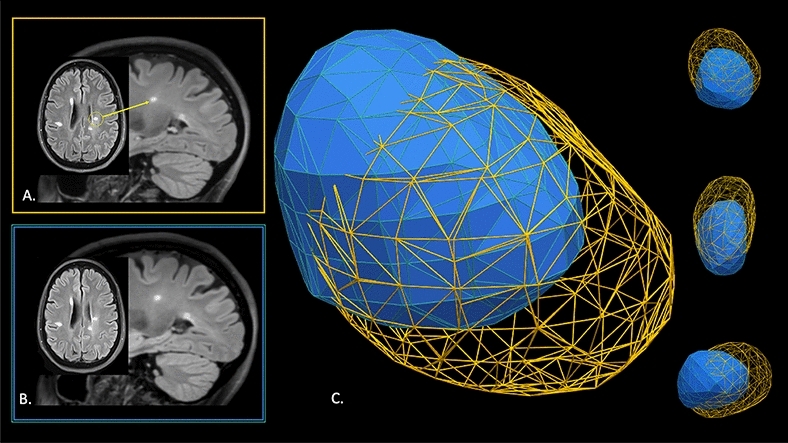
Two-dimensional (2D) MRI axial FLAIR and 3-dimensional (3D) sagittal FLAIR images highlighting a single MS lesion (circled in yellow) from a 49-year-old white woman with relapsing remitting disease from (A) time point 1 and (B) time point 2 (1-year follow-up). (C) Visual model in 3D demonstrating displacement and deformation of the lesion at time point 2 (blue) compared to the original position at time point 1 (yellow mesh). Note both the reduction in size and positioning of the lesion between time points that is not apparent on review of the longitudinal 2D MRI data (A,B).
